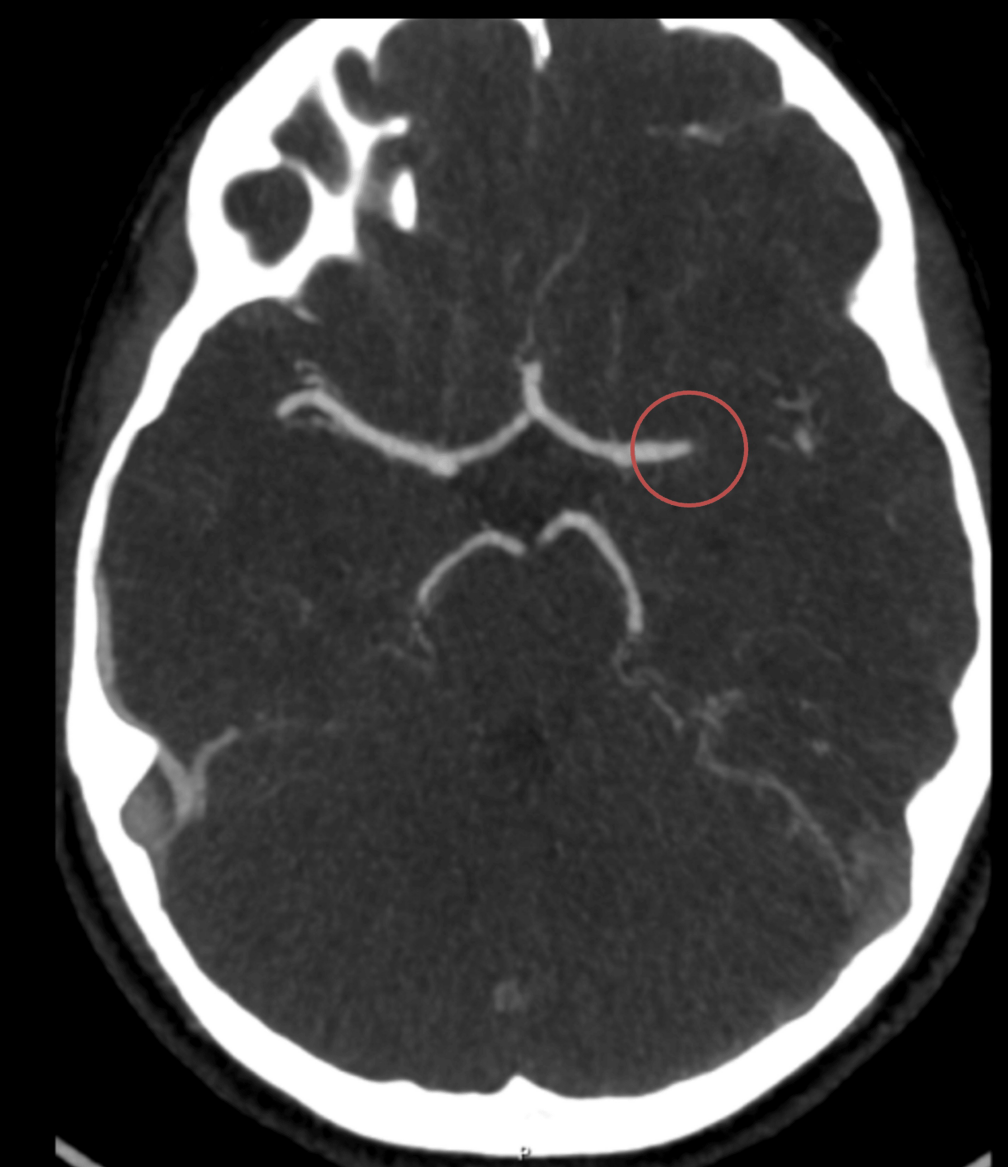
CTA shows occlusion of the first segment of the MCA CTA: computerized tomography angiography; MCA: middle cerebral artery.
